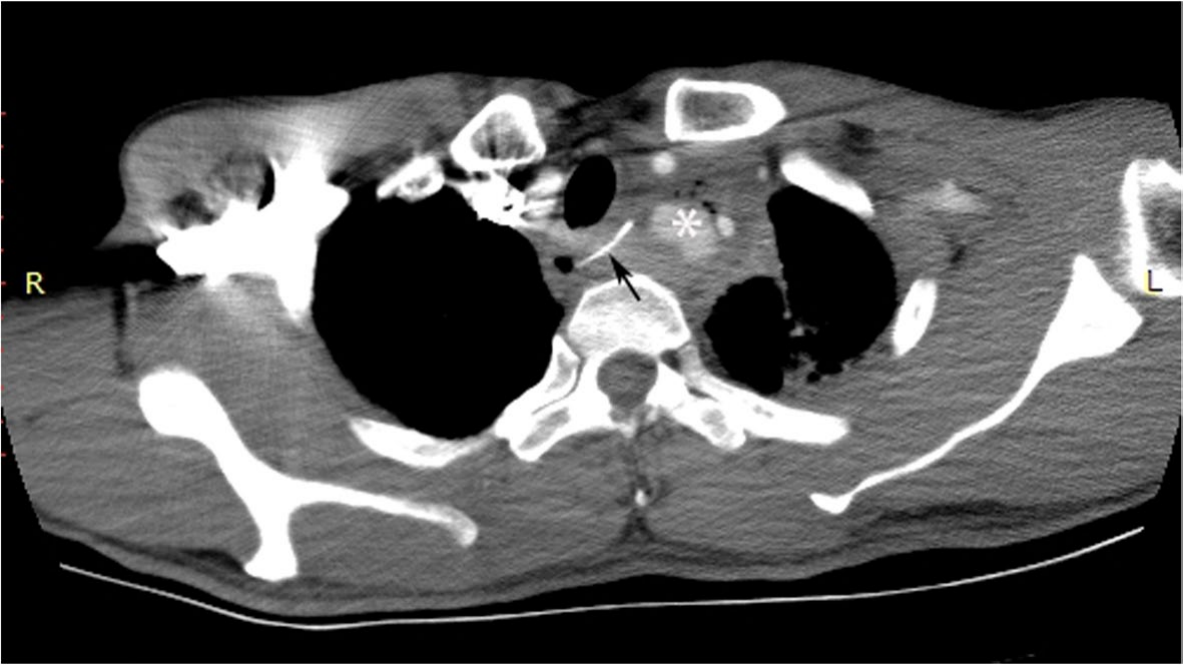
Enhanced computed tomography showed a fish bone that had penetrated the left superior mediastinum through the esophagus. An aneurysmal hematoma had formed because the left subclavian artery was pierced. Exudation is present in the mediastinum around esophagus and left pleural cavity. Black arrow: fish bone; White star: aneurysmal hematoma enhanced by contrast medium
